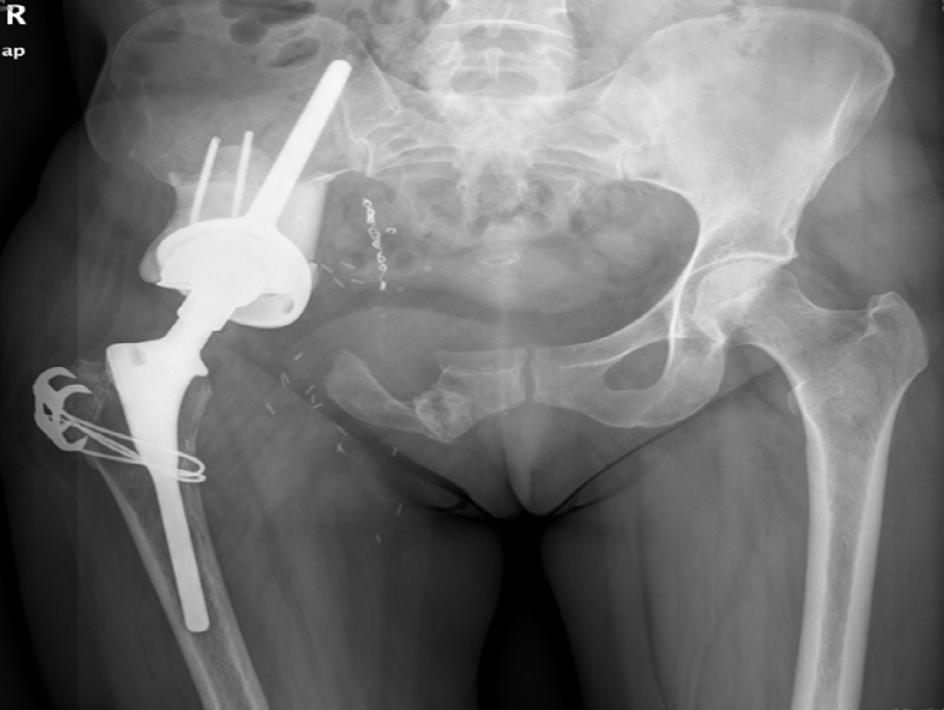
Radiographic result at 3-year follow-up.
